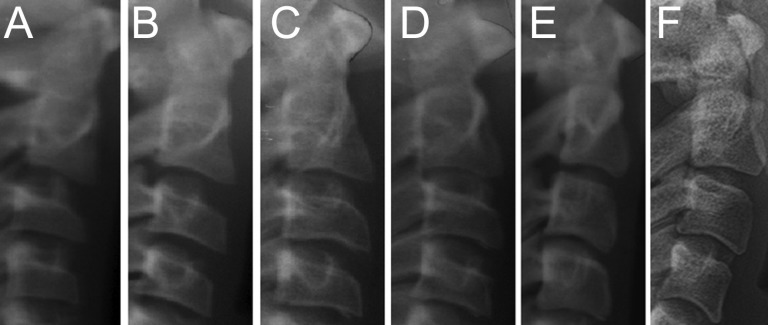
Radiographs of the six skeletal stages proposed by Hassel and Farman (9) for the development of the cervical vertebrae C2, C3 and C4.
